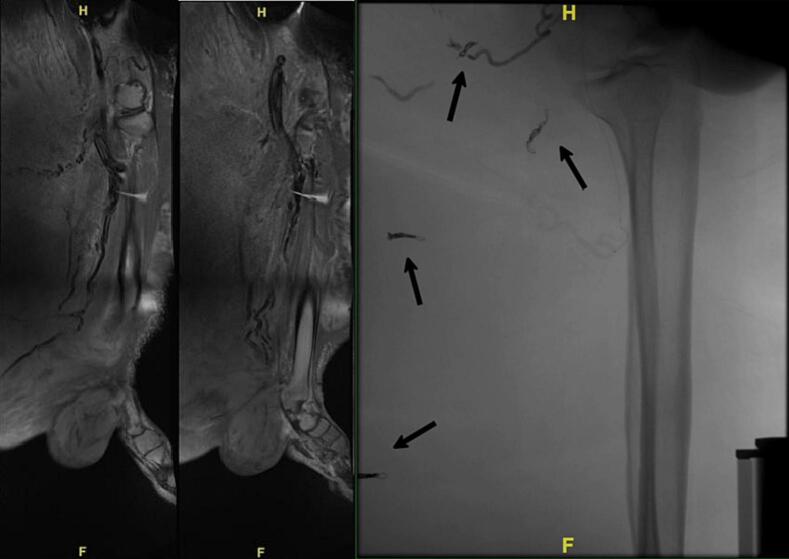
(A) MRI of the patient's left lower extremity, showing a mass infiltrating the gastrocnemius, soleus, peroneus longus, and brevis muscles. Hypervascularization was shown surrounding the mass in close proximity to important vessels of the lower extremity. (B) Embolization of the feeding branches of the popliteal and peroneal arteries. A total of four branches were embolized, shown by the straight arrows.
